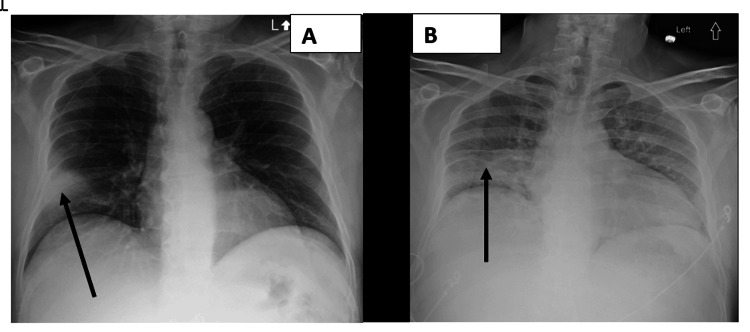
Chest X-rays taken on admission (A) and on day 6 of hospitalization (B). Black arrows indicate areas of consolidation in the right middle-to-lower zones. Both images show no evidence of lung abscess, empyema, or pleural effusion
